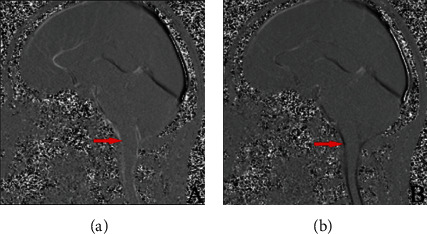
PC-MRI images ((a) high signal indicates downward movement of systolic CSF (as indicated by the red arrow); (b) low signal indicates headward movement of diastolic CSF (as indicated by the red arrow)).
